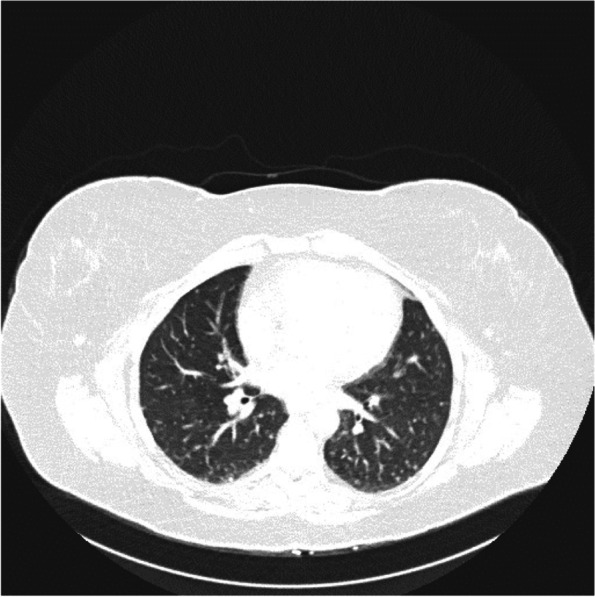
Imaging findings. Selected axial non-contrast chest CT image shows bilateral multiple small nodules randomly distributed through both lungs
